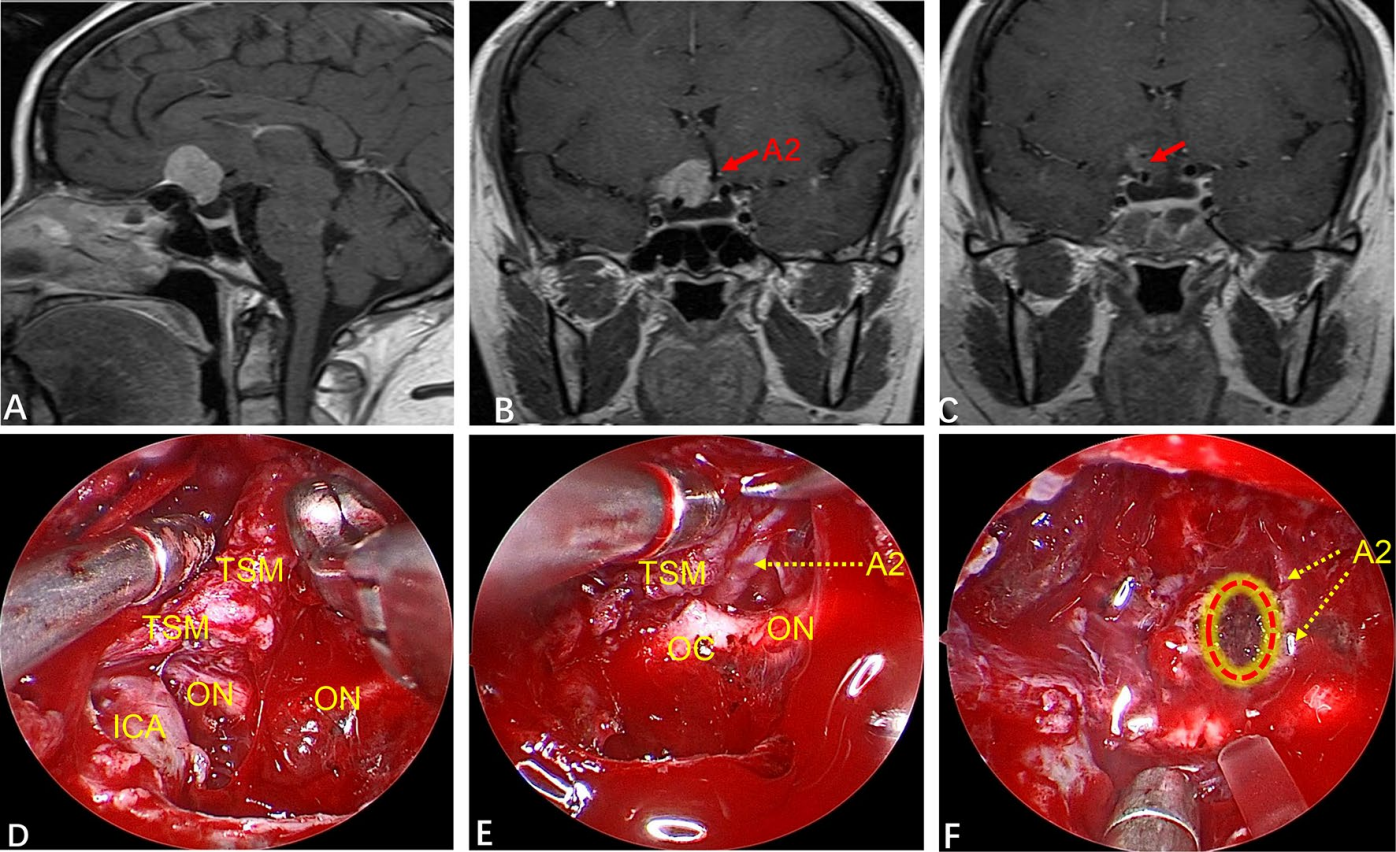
(A,B) Contrast-enhanced MRI preoperatively indicated that the tumor was closely related to the internal carotid artery and A2 branch of ACA. (C) At POD3, MRI reexamination showed the degree of tumor resection. There was no tumor residue at the A2 branch of the ACA. Whether there was a small amount of tumor remaining at the right internal carotid artery (ICA) was not clear (red arrow). (D) This intraoperative image shows the tumor mass toughened by fibrosis and beyond the right ICA. (E) There was a close relationship between the A2 branch of the ACA and fibrosis-toughened tumors. (F) A few tumors were linked inextricably to the A2 branch of the ACA, and residual tumors were electrocoagulated repeatedly intraoperatively (red dotted circular box).
